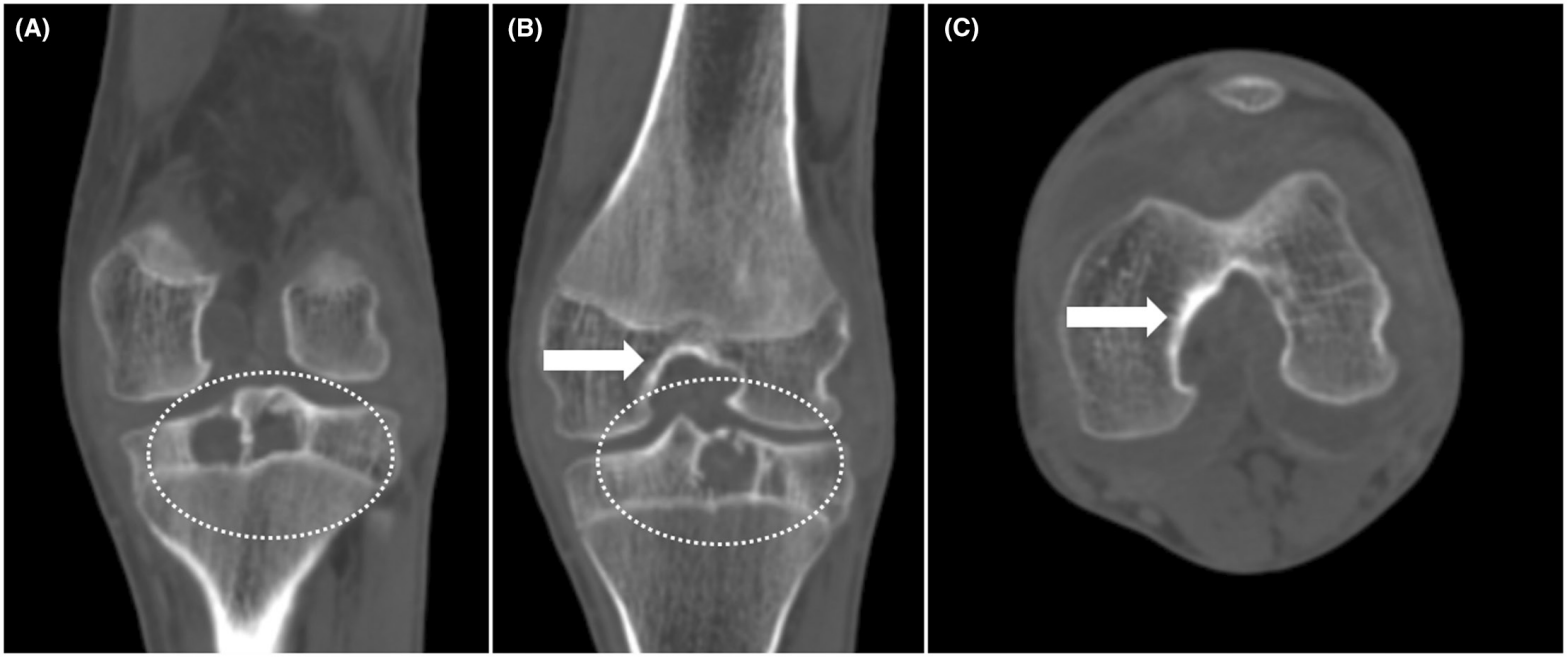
Coronal reconstructed (A, B) and axial (C) non contrast CT images of knee joint showing lytic lesions in tibial plateau (dotted circles) and widened intercondylar notch (block arrows).
